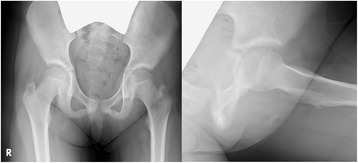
Patient radiographs on admission. Anteroposterior (*left*) and lateral (*right*) radiographs showing no obvious osseous abnormality
